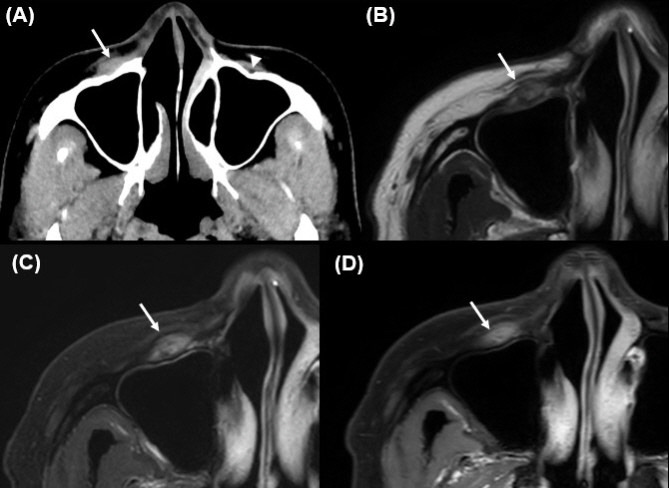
Cross-sectional images, including CT and MRI. (A) Contrast-enhanced axial CT image shows a mildly enhancing focal soft tissue lesion (arrow) in the right premaxillary fat pad, involving the ipsilateral infraorbital foramen (arrowhead; contralateral side shown for comparison). (B–D) Axial MR images demonstrate the lesion as mixed low to isointensity on T1-weighted imaging (B), inhomogeneous hyperintensity with focal linear hypointense foci on fat-suppressed T2-weighted imaging (C), and mild to moderate heterogeneous enhancement relative to adjacent muscle and fat planes on contrast-enhanced fat-suppressed T1-weighted imaging (D). The lesion extends into the juxtaforaminal fat pad and partially involves the levator labii superioris muscle. These findings, including proximity to and suspected involvement of the infraorbital nerve, raised concern for locoregional tumor recurrence in a patient with prior adenoid cystic carcinoma.
